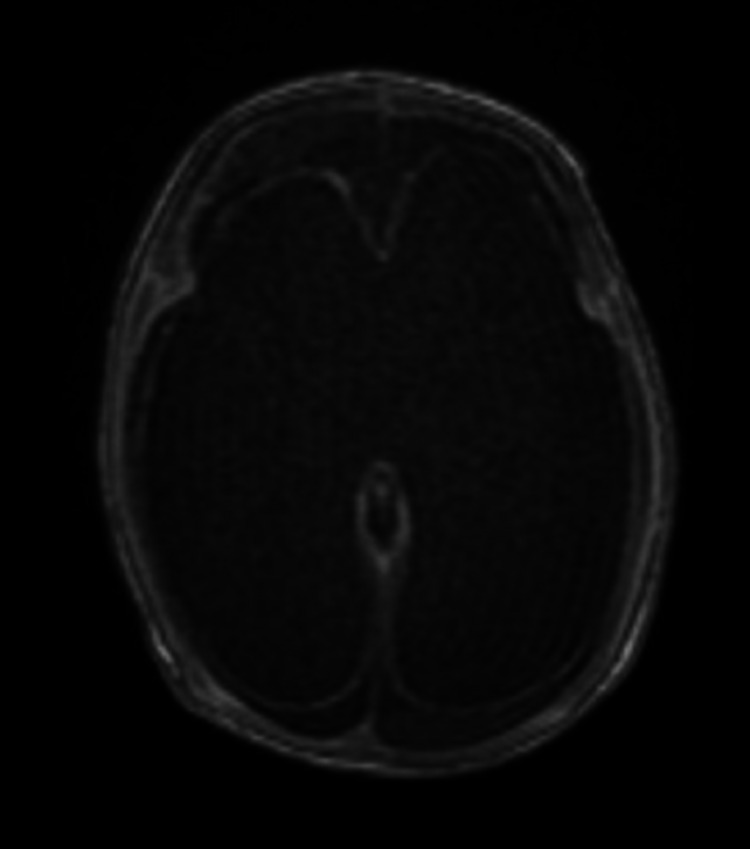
Postnatal brain magnetic resonance imaging of a patient with severe brain volume loss and mantel index thinning
